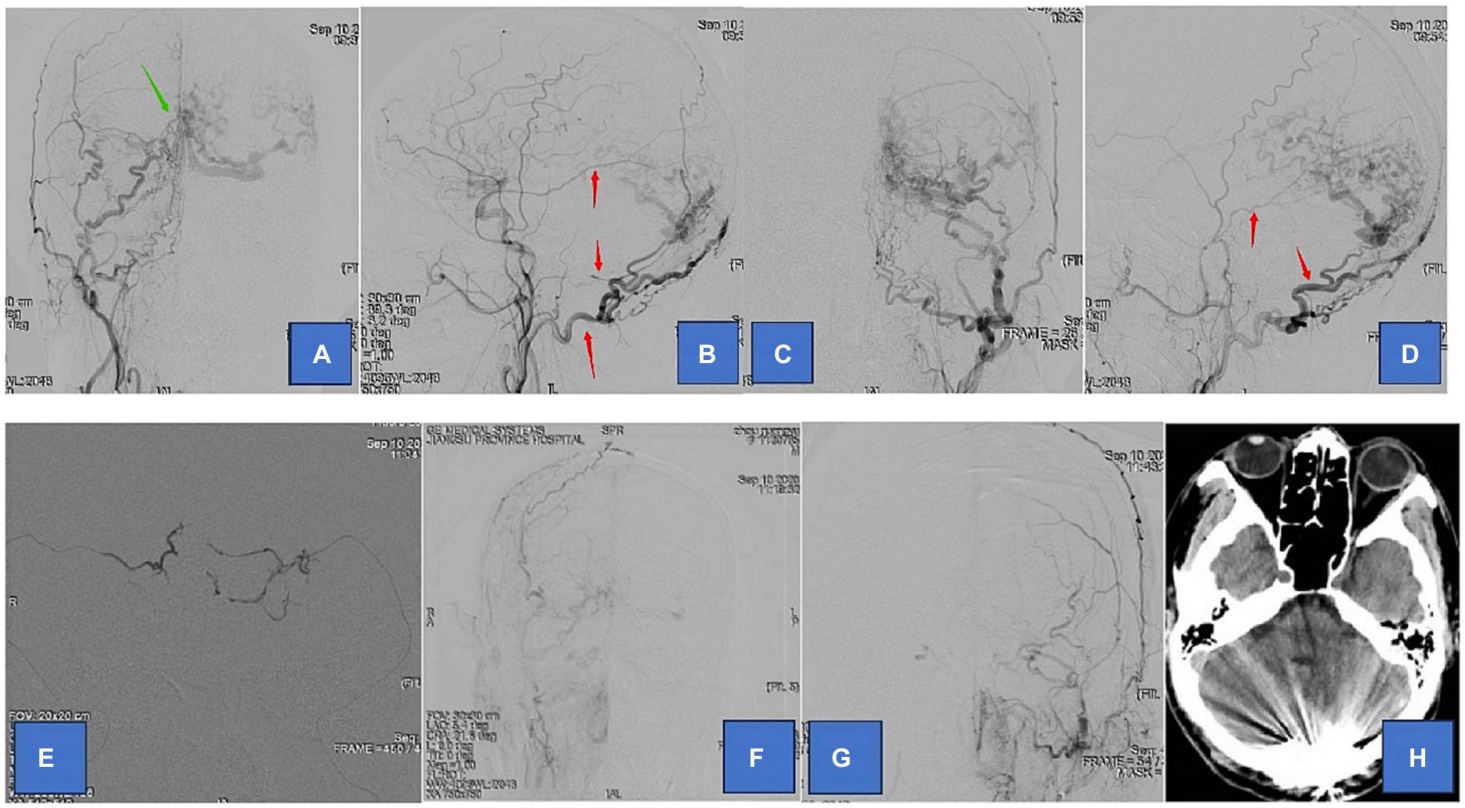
Adult patient presented with headache for 1 month, whose DSA suggested a tentorial dural arteriovenous fistula of type G3. (A,B) are the frontal and lateral views of the right external carotid artery, showing the right occipital, middle meningeal, and ascending pharyngeal arteries involved in the blood supply. (C,D) are the frontal and lateral views of the left external carotid artery showing the left occipital, middle meningeal, and posterior auricular arteries involved in the blood supply. (E) We chose the bilateral middle meningeal artery route for the embolization of TDAVF. (F,G) are postoperative bilateral external carotid arteriograms showing complete embolization of the TDAVF. (H) is a postoperative cranial CT showing hyperdense embolic shadow in the sinus convergence area. Red arrows point to arteries, and green arrows point to TDAVF.
